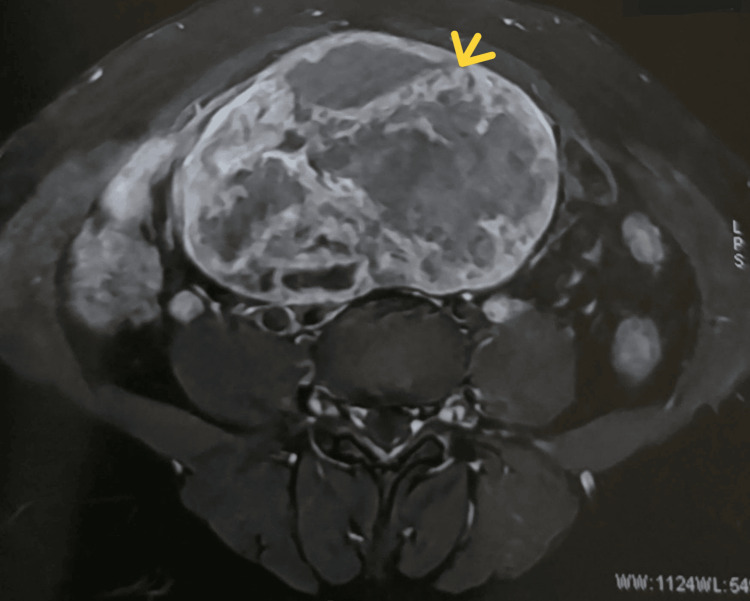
MRI pelvis Axial MR image showing a large 15 x 12 x 11 cm heterogeneous, well-circumscribed uterine mass (yellow arrow), which is irregularly thick, lobulated, heterogeneously enhancing solid lesion with central necrotic cystic areas arising from fundal and posterior wall of uterus
